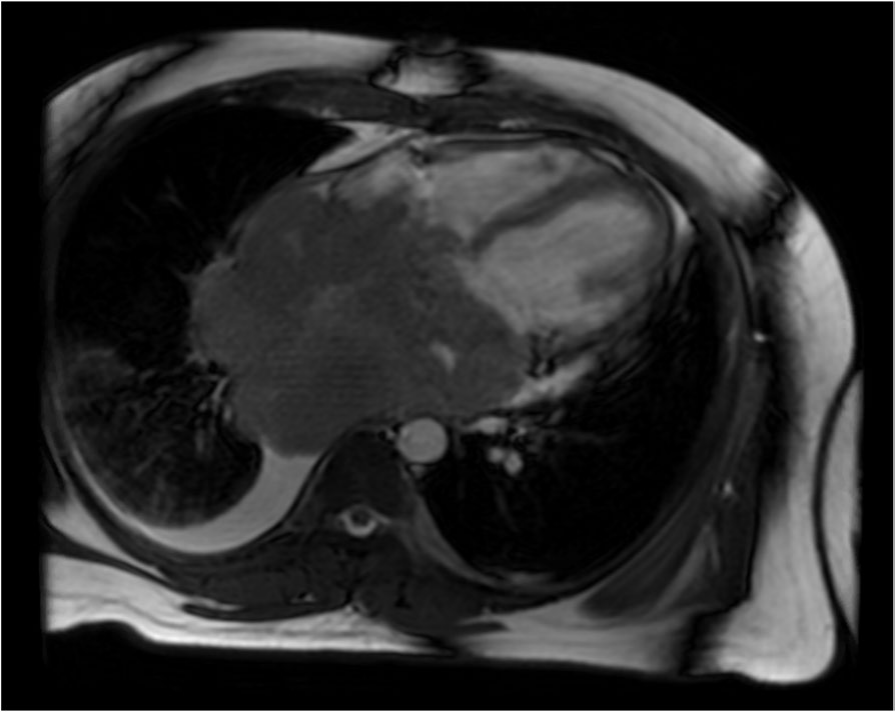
Cardiac MRI with contrast showing neoplasm with biatrial and pericardial invasion, approximately 15 cm in diameter
